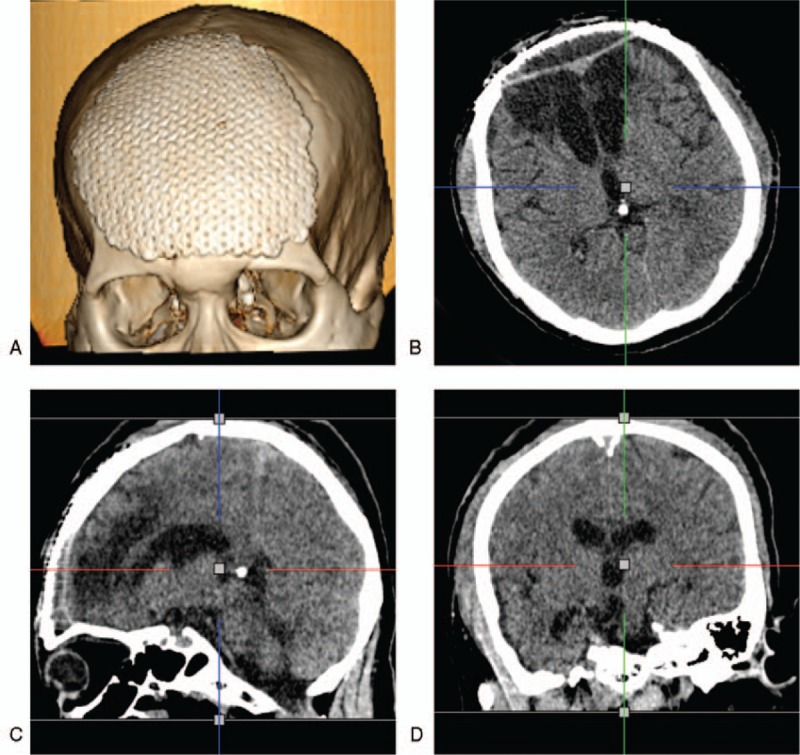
CT images after cranioplasty. A–D: 3D reconstruction and thin-slice CT showed the appropriate positioning of the metal titanium plate and normal brain ventricles. CT = computerized tomography.
